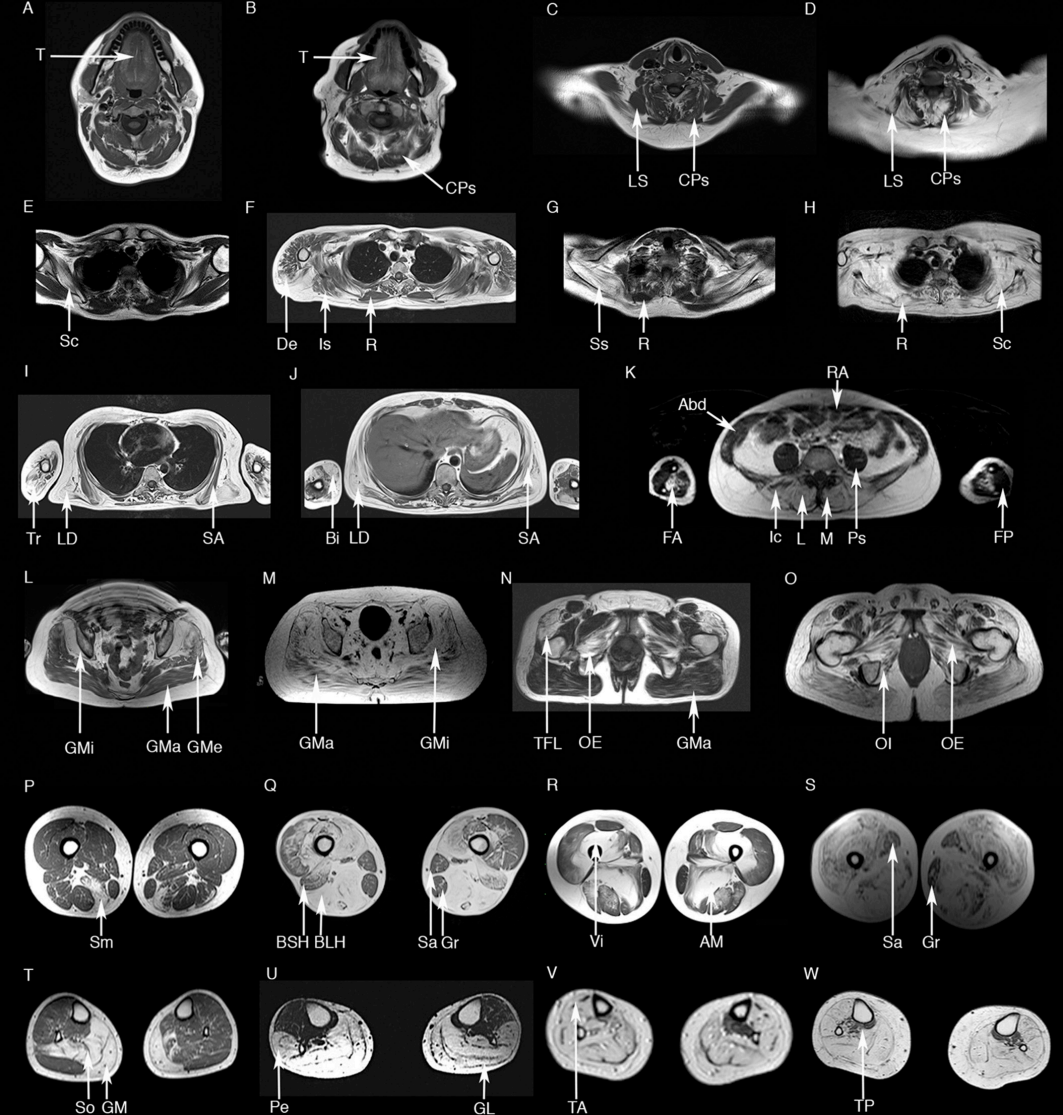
Axial T1-weighted muscle MRI in dysferlinopathy. The cranial muscles (A–B) more commonly replaced by fat are the tongue (T) and the cervical paraspinal muscles (CPs). *Levator scapulae* (LS) is generally not involved until later stages of the disease (C, no involvement; D, involvement). In the scapular region (E–H) the *subscapularis* (Sc) is involved at the early stages; other muscles such as *deltoid* (De), *infraspinatus* (Is) or *supraspinatus* (SS) become progressively involved. *Rhomboideus* (R) tends to be not involved until later stages of the disease (E–G, no involvement; H, involvement in an advanced case). *Biceps brachii* (Bi), *triceps brachii* (Tr) and the anterior muscles of the forearm (FA) are commonly involved (I–K), while the posterior muscles of the forearm (FP) are not involved even in later stages of the disease. *Latissimus dorsi* (LD) tends to be involved before *serratus anterior* (SA) in most of the patients (I–J). Paraspinal muscles including the *multifidus* (M), the *longissimus* (L) and the *iliocostalis* (Ic) are affected in most of the patients at symptom onset, while abdominal muscles, such as *rectus abdominis* (RA) are more resistant and become affected only in latter stages (K). *Gluteus minor* (GMi) is more severely involved than *gluteus medius* (GMe) and *maximus* (GMa) (L–M). Pelvic floor muscles are transformed by fat in patients with dysferlinopathy, with the *tensor fascia latae* (TFL), *obturatorius externus* (OE) and *internus* (OI) being the muscles more commonly involved (N–O). The posterior muscles of the thighs (*semimembranosus* (Sm), *biceps femoris long head* (BLH), *biceps femoris short head* (BSH) or *adductor major* (AM)) are commonly involved in most of the patients (P–S). BSH tend to be less involved than BLH in early and mid-stage patients (Q). *Vasti* muscles are commonly involved even in early stages of the diseases (*vastus intermedius* (VI) in R). In contrast *Sartorius* (Sa) and *gracilis* (Gr) are not involved until late stages of the disease (Q and S). Analysis of the lower legs (T–W) shows initial involvement of *gastrocnemius medialis* (GM) and *lateralis* (GL) and *soleus* (So). *Peroneus* muscles (Pe) are also involved in most of the patients (U). Later in the progression of the disease *tibialis anterior* (TA) and *posterior* (TP) become transformed by fat (V–W).
